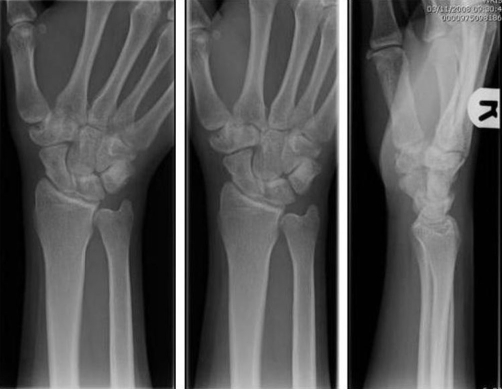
Check X-rays of right hand at 3 month follow-up.
